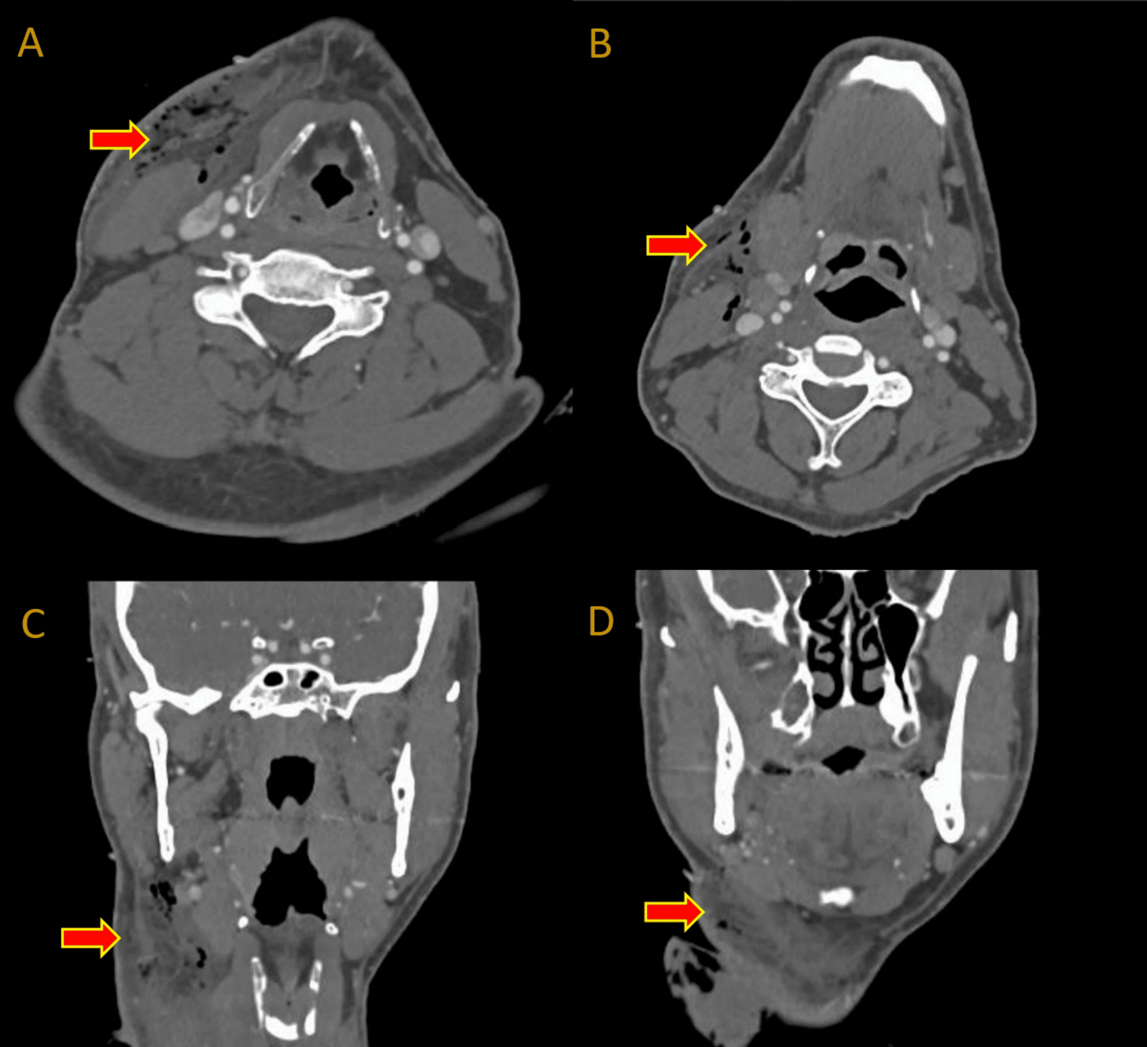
A preoperative contrast-enhanced computed tomography scan of the neck. Axial slices (panes A and B) and coronal slices (panes C and D) with arrows highlighting areas of surgical emphysema, disorganised haematoma, and paint infiltration of superficial and deep cervical tissue planes.
